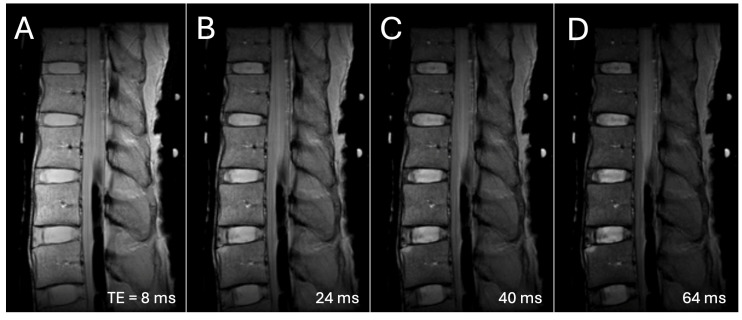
Representative sagittal MR images of a lumbar spine acquired at varying echo times (TE) of (A) 8 ms, (B) 24 ms, (C) 40 ms, and (D) 64 ms.
